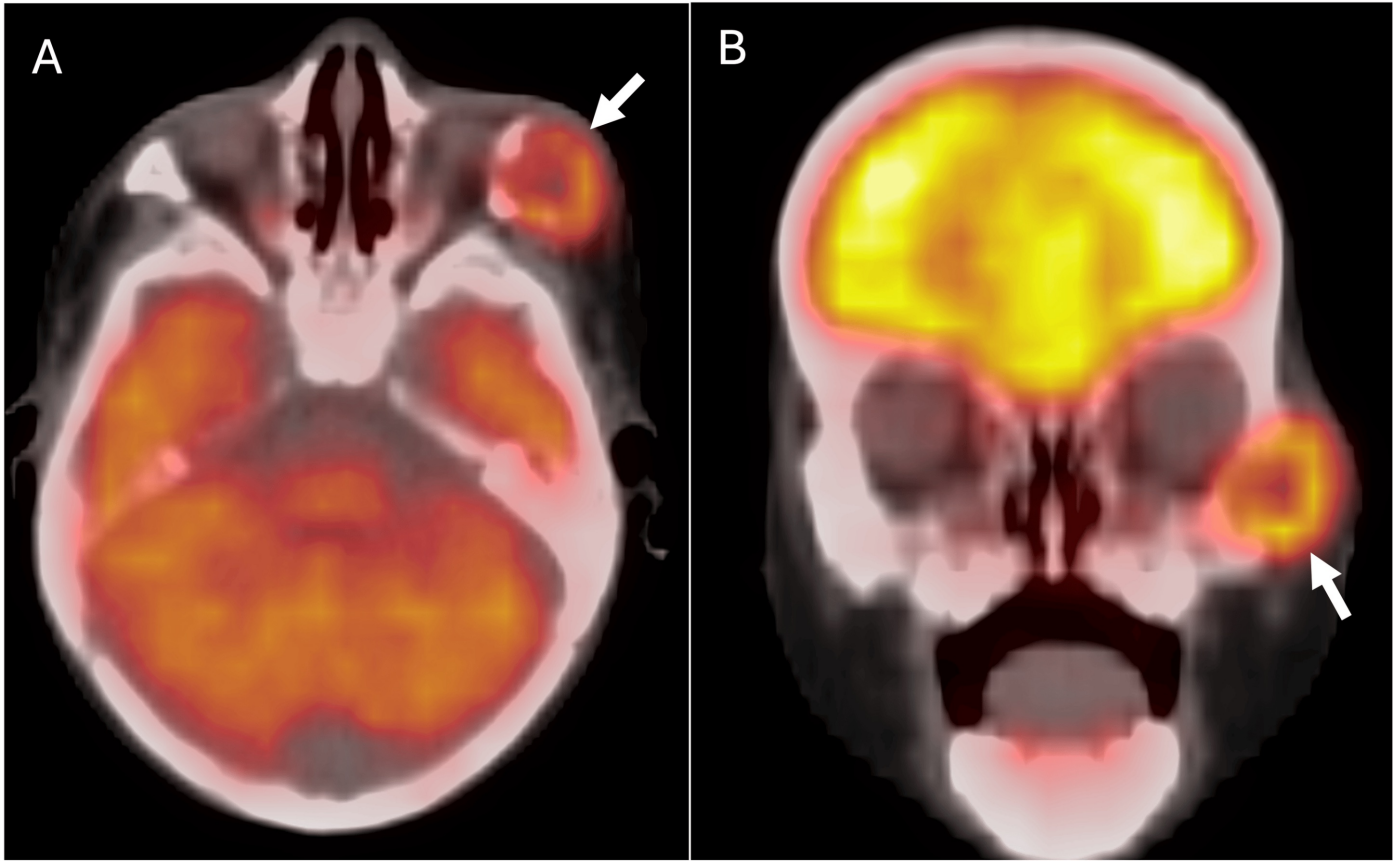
Axial (A) and coronal (B) fused FDG PET/CT images show moderate hypermetabolism (arrow) corresponding to the expansile septated left zygomatic mass.
